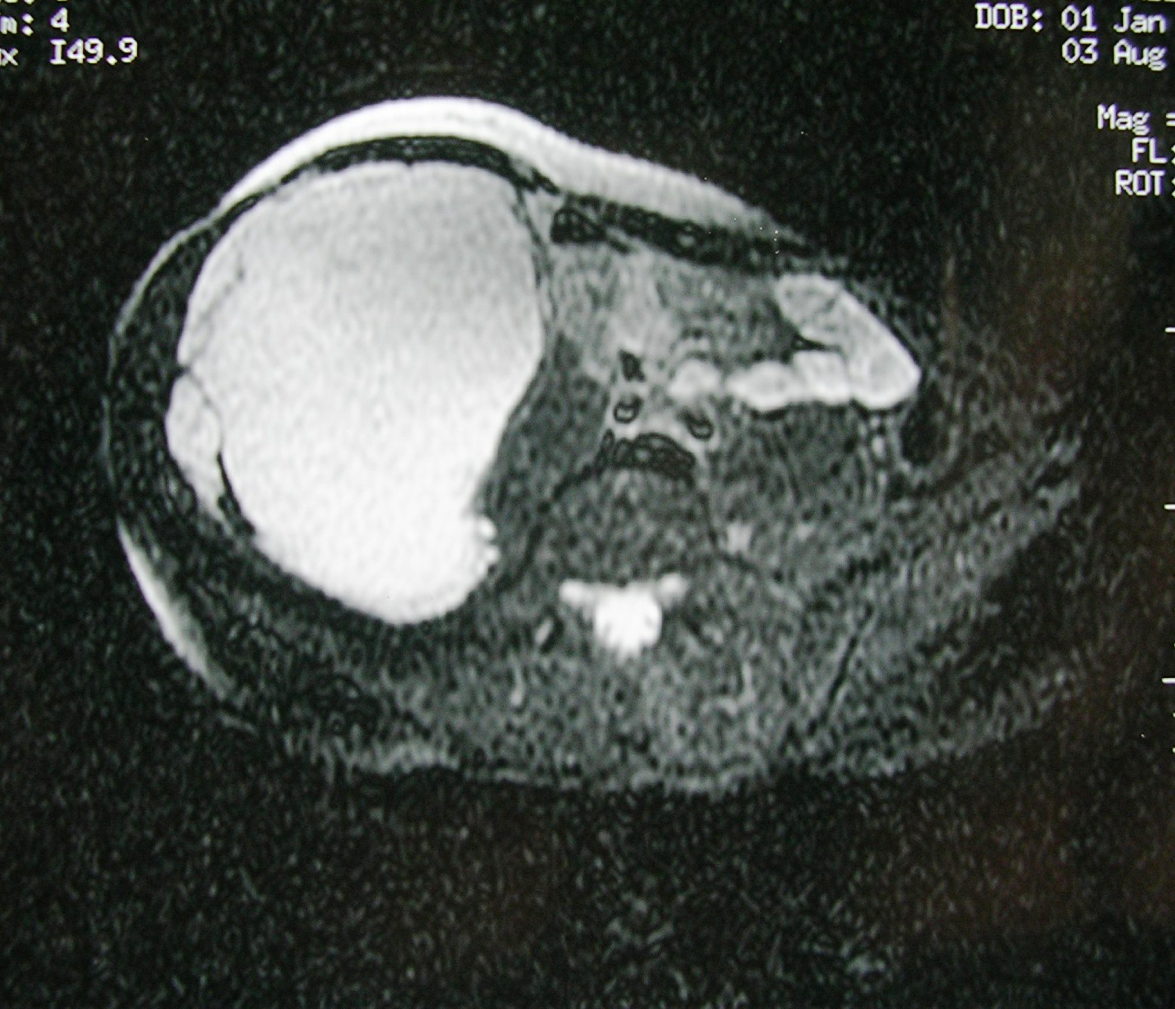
Axial T2 MR image with fat suppression revealing the cystic character of the tumor.
